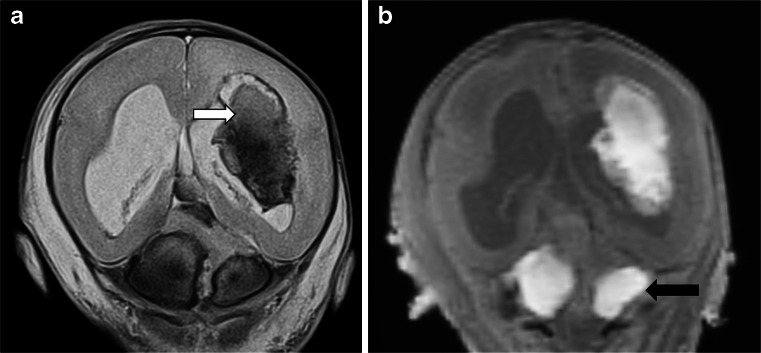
Intracranial haemorrhage. Coronal T2-weighted (a) postmortem magnetic resonance imaging imaging of the brain at 25 weeks, showing haemorrhage in the left lateral ventricle with parenchymal haemorrhage (*white arrow*), and blood in the basal cistern (*black arrow*), which is high signal on T1-weighted images (b). This degree of haemorrhage is clearly pathological, but small intraventricular haemorrhages in fetuses may occur spontaneously
